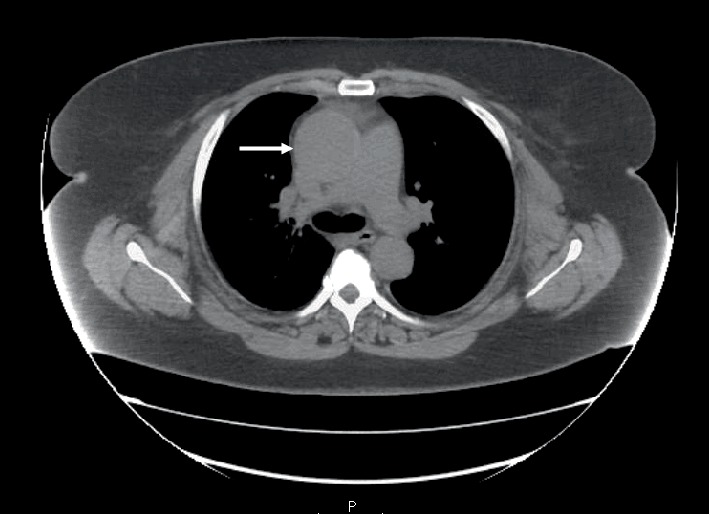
Axial CT image of the thorax without contrast showing aneurysmal dilatation (arrow) of the ascending thoracic aorta.
